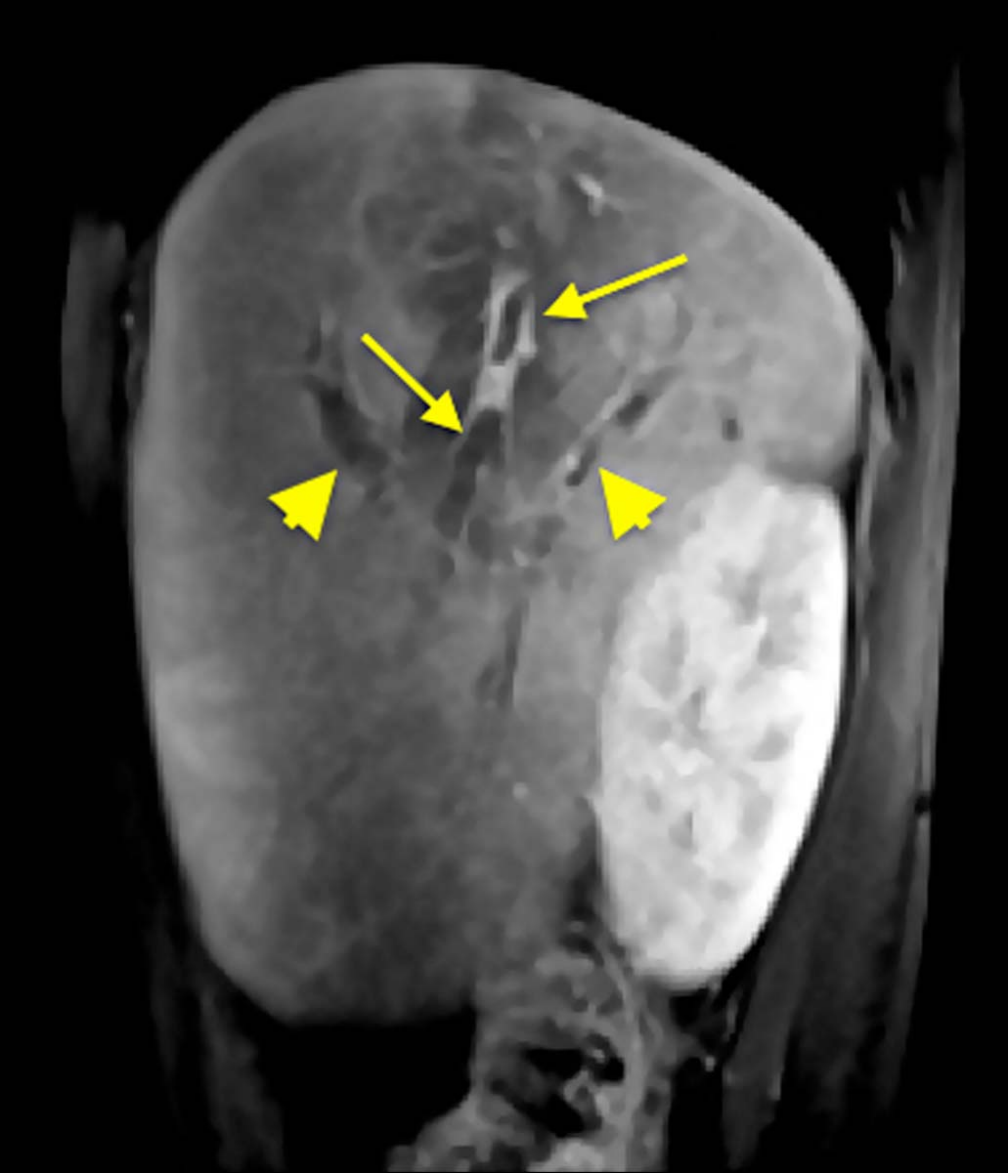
Sagittal view of abdominal MRI showing filling defects within the right hepatic vein (arrows) and right portal veins (arrowheads).
